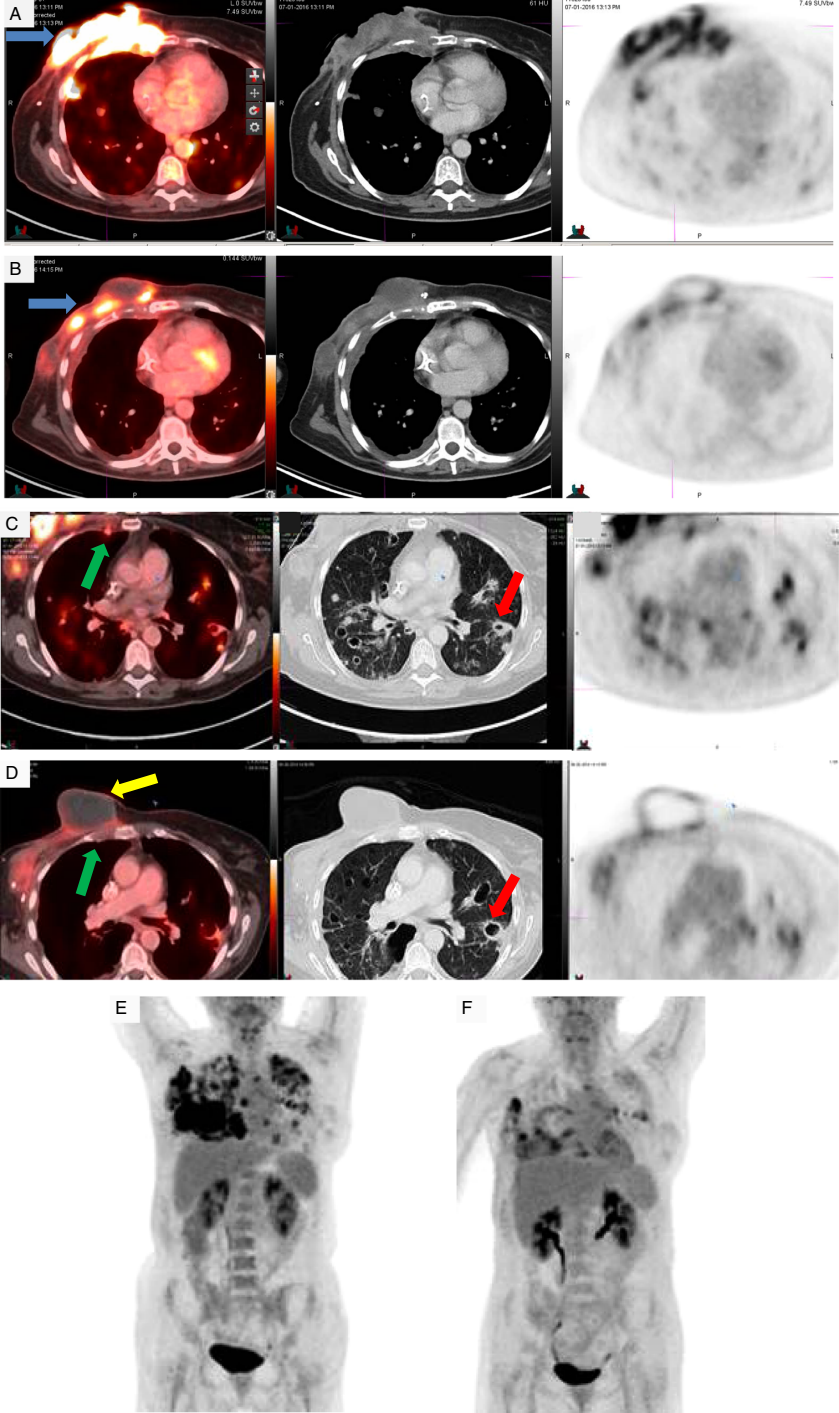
Radiographic response of recurrent metaplastic breast cancer with a bulky chest wall mass and pulmonary metastases to pembrolizumab/nab-paclitaxel. PET CT images a at baseline depicting the large and destructive chest wall mass (SUV 11.0, 10.1 × 3.6 cm, *blue arrow*), invading ribs, sternum, and pleura, b at 9 weeks (s/p three cycles) demonstrating a significant metabolic and anatomic response on the chest wall mass (SUV 5.6, 6.5 × 4.7 cm, *blue arrow*), c at baseline depicting bilateral lung parenchymal (*red arrow*) and nodal metastases (*green arrow*), d at 9 weeks demonstrating a decreased number, size, and metabolic activity of pulmonary metastases and worsening cavitation (*red arrow*) as sign of favorable response to therapy. Additionally, the protruding mass (seen on the clinical photographs) is shown with necrotic debris. Complete response to the previously identified intensely FDG avid necrotic right internal mammary chain lymph node (*green arrow*). e Coronal image at baseline depicting the bulky right chest wall recurrence, extensive bilateral lung parenchymal and pleural metastases as well as nodal metastases. f Coronal image at 9 weeks demonstrating marked interval improvement in the right chest wall mass consistent with a partial treatment response, as well as interval decrease of multiple FDG avid nodal, pulmonary, and pleural metastases
